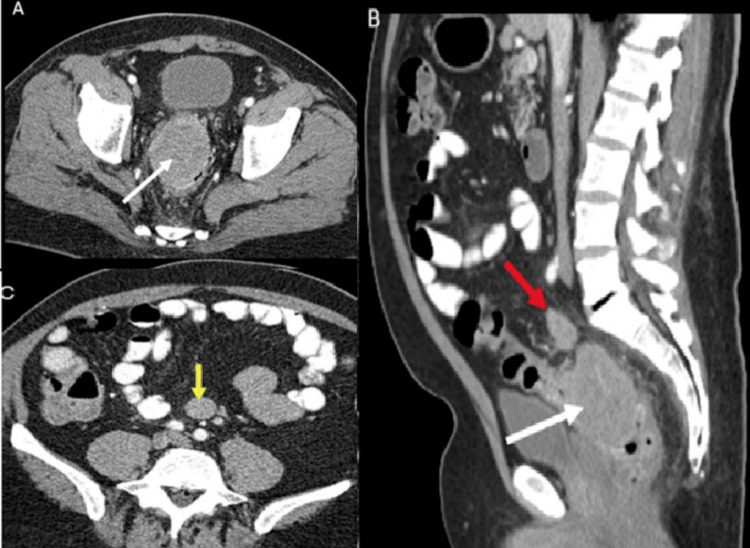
Preoperative CT scan of the abdomen and pelvis Axial (A) and sagittal (B) contrast-enhanced images of the abdomen and pelvis showing a large mass (7 x 5 cm; white arrows) centered in the right side of the proximal rectum. Note the nodal mass (red arrow) located just superior to the rectosigmoid mass. An axial image (C) obtained more superiorly shows an additional nodal mass (yellow arrow) in the lower retroperitoneum CT: computed tomography
